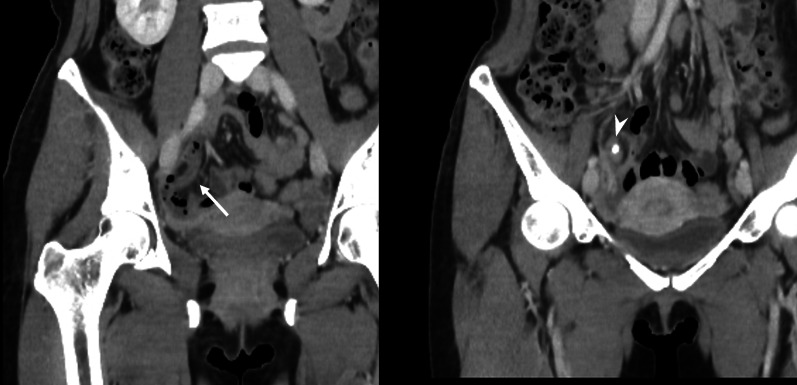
Abdominal contrast-enhanced computed tomography findings after conservative treatment. The wall thickness and swelling of the appendix (white arrow) were improved. The fecalith (white arrowhead) remained near the root of the appendix
